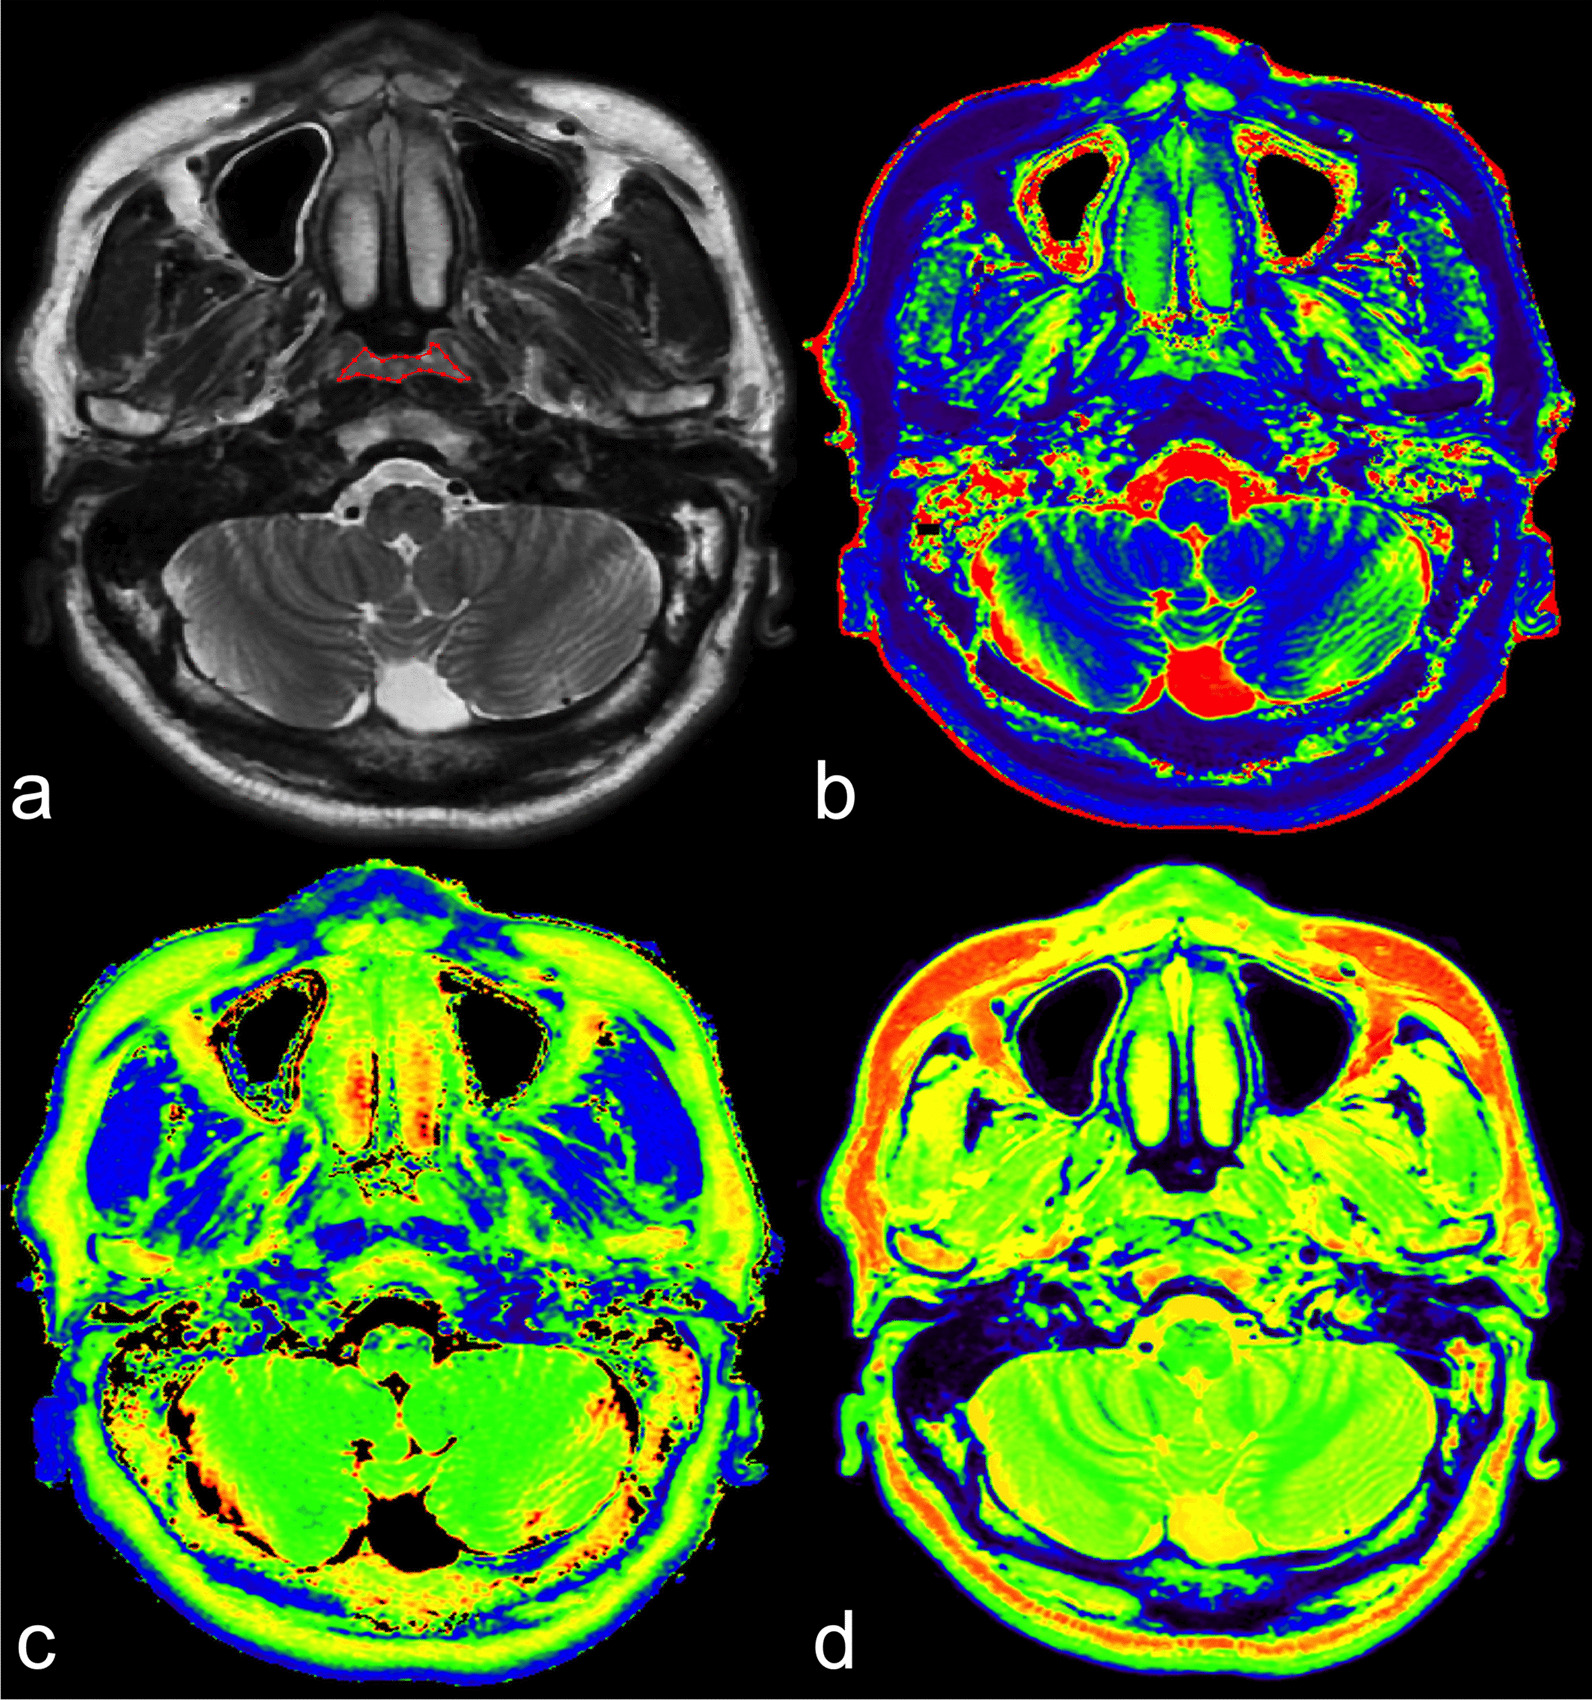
SyMRI of a 48-year-old male NPC patient. a Axial T2WI shows uniform thickening with heterogeneous iso-slightly high signal in the posterior wall of the nasopharyngeal cavity, and bilateral pharyngeal recess are collapsed. VOI was manually delineated along the border of the tumour slice by slice. T1 map (b), T2 map (c) and PD map (d) at the same level as in (a). SyMRI, synthetic magnetic resonance imaging; NPC, nasopharyngeal carcinoma; T2WI, T2-weighted image; VOI, volume of interest; PD, proton density
